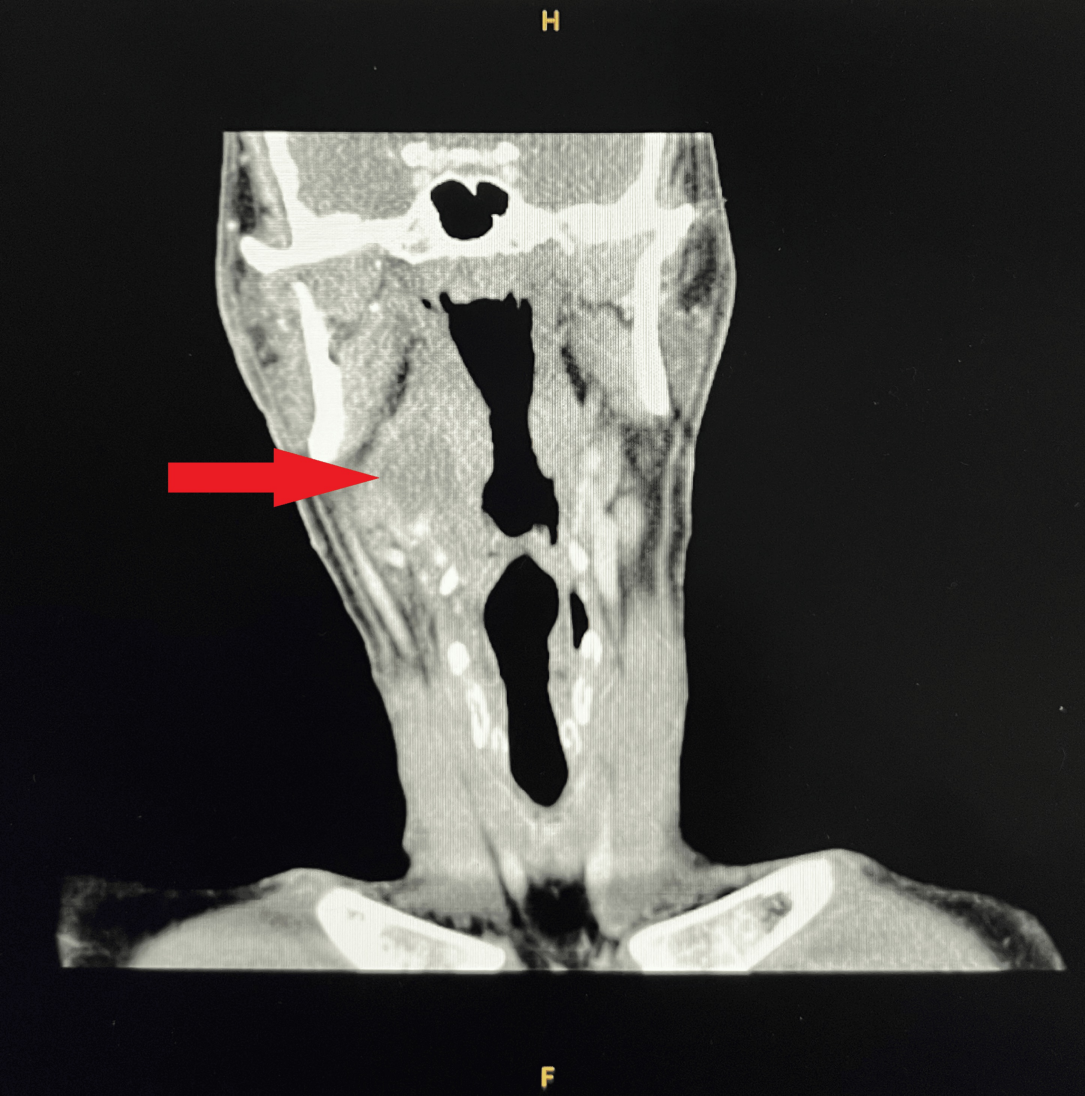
Computed tomography (CT) of the neck showing an abnormal tonsillar pathology, indicated by a red arrow.
